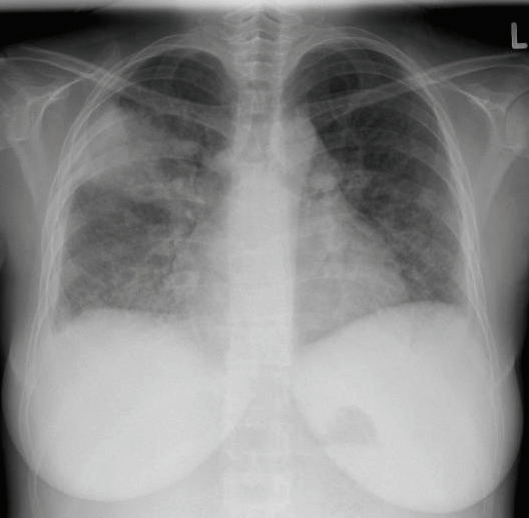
Chest X-ray on admission day.
